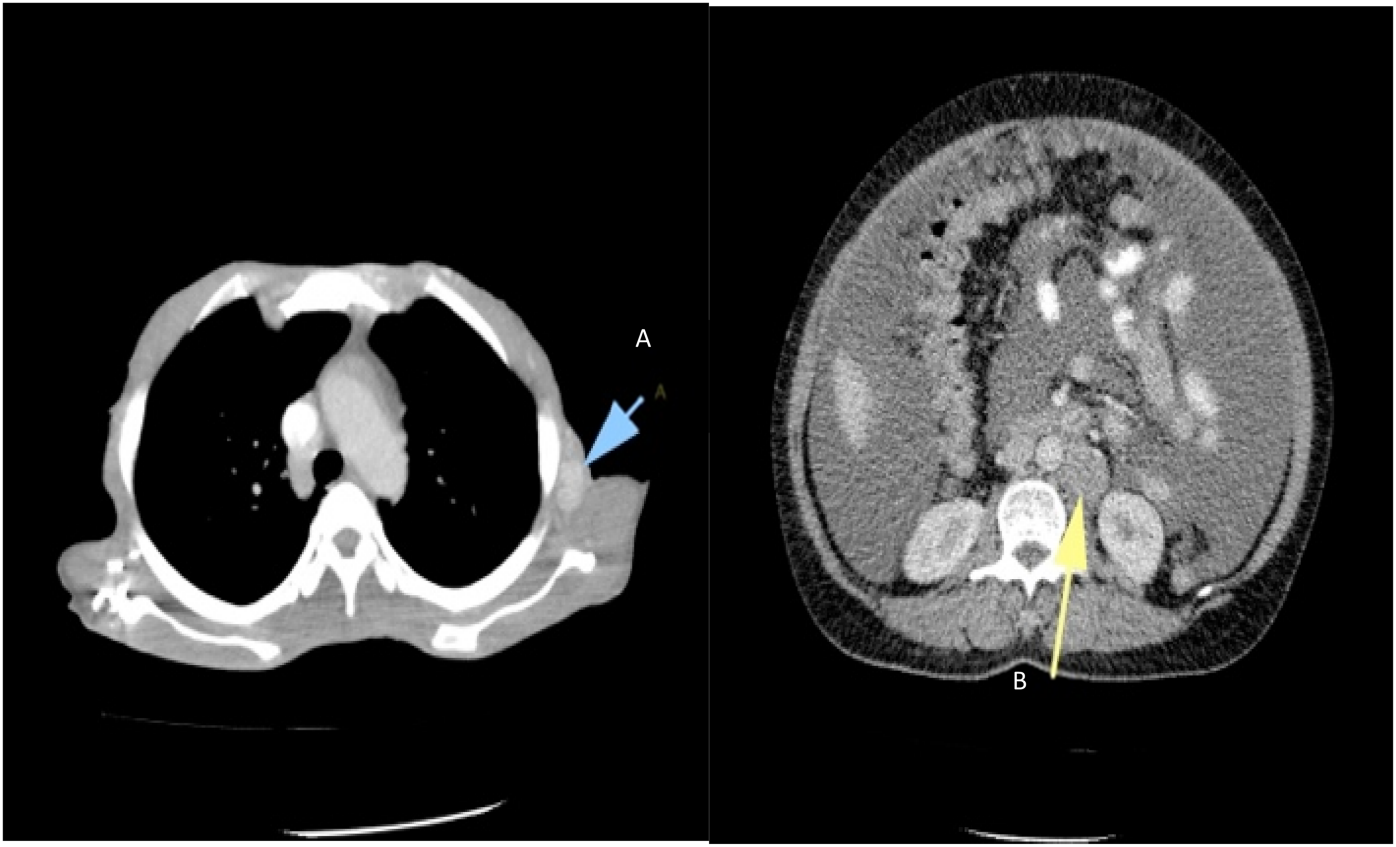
Axial computed tomography scan of the abdomen and chest shows enlarged round/oval lymph nodes in the axilla (A) and portal hepatis (B).
